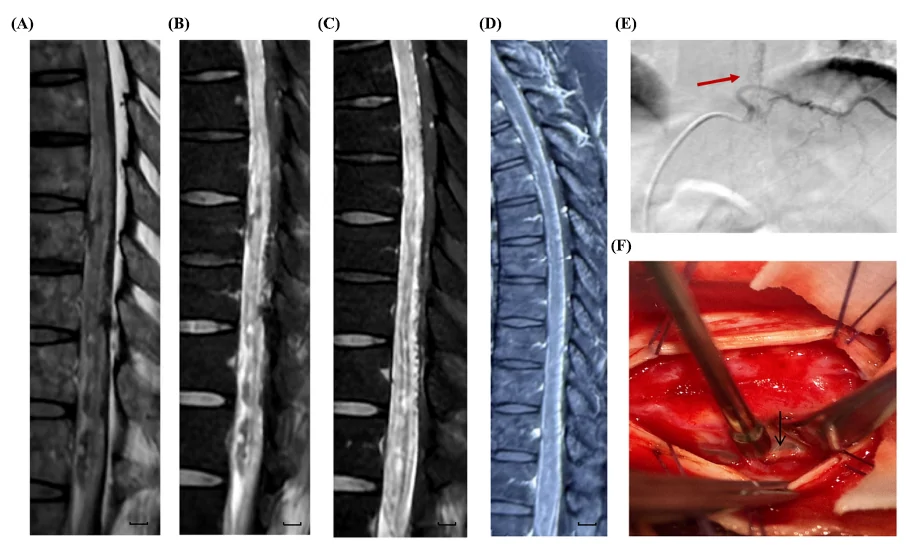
Spinal cord MRI and spinal angiography. A. MRI of the thoracic spine revealed multiple patchy high T2WI signals in the thoracic spinal cord and hemosiderin deposition in the dorsal spinal cord; B. Multiple curved vascular structures around the spine on fat-suppression sequence; C. Irregular thickening and enhancement of the spinal meninges; D. On angiography of the left T10 intercostal artery; E. The dural veins were early and tortuous and dilated (red arrow); F. Intraoperative findings of arterialized veins proximal to the fistula (white arrow). MRI, magnetic resonance imaging; T2WI, T2-weighted imaging. Scale bars = 10 mm.
